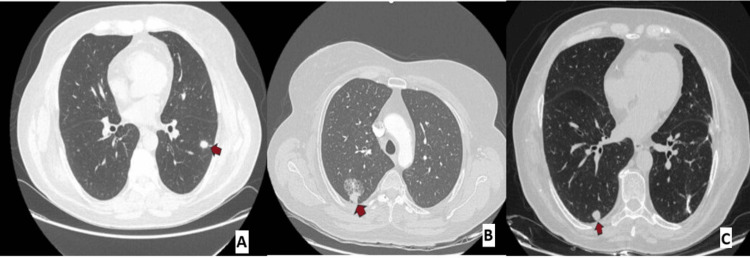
Thoracic computed tomography (CT) showing the patient's lesions over the years (highlighted by red arrows) A: 13 mm nodule in the lower lobe of the left lung on thorax CT, B: 34 mm ground-glass lesion with a solid component in the posterior upper lobe of the right lung on thorax CT, C: 10 mm solid nodule in the posterior lower lobe of the right lung on thorax CT
